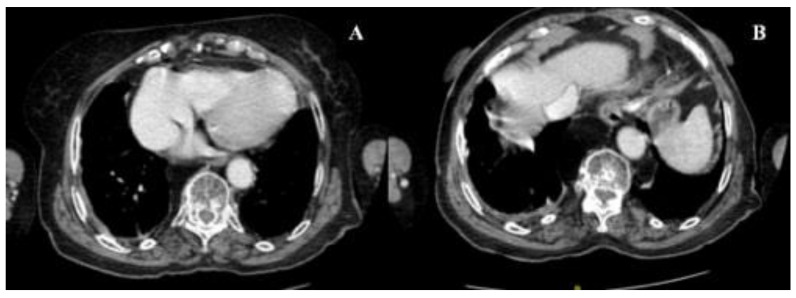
Thoracic-abdominal-pelvic CT, performed after eight cycles of chemotherapy, showing radiological improvement, without lymphomatous masses in right superior lobe (A) or right pleural effusion (B).
